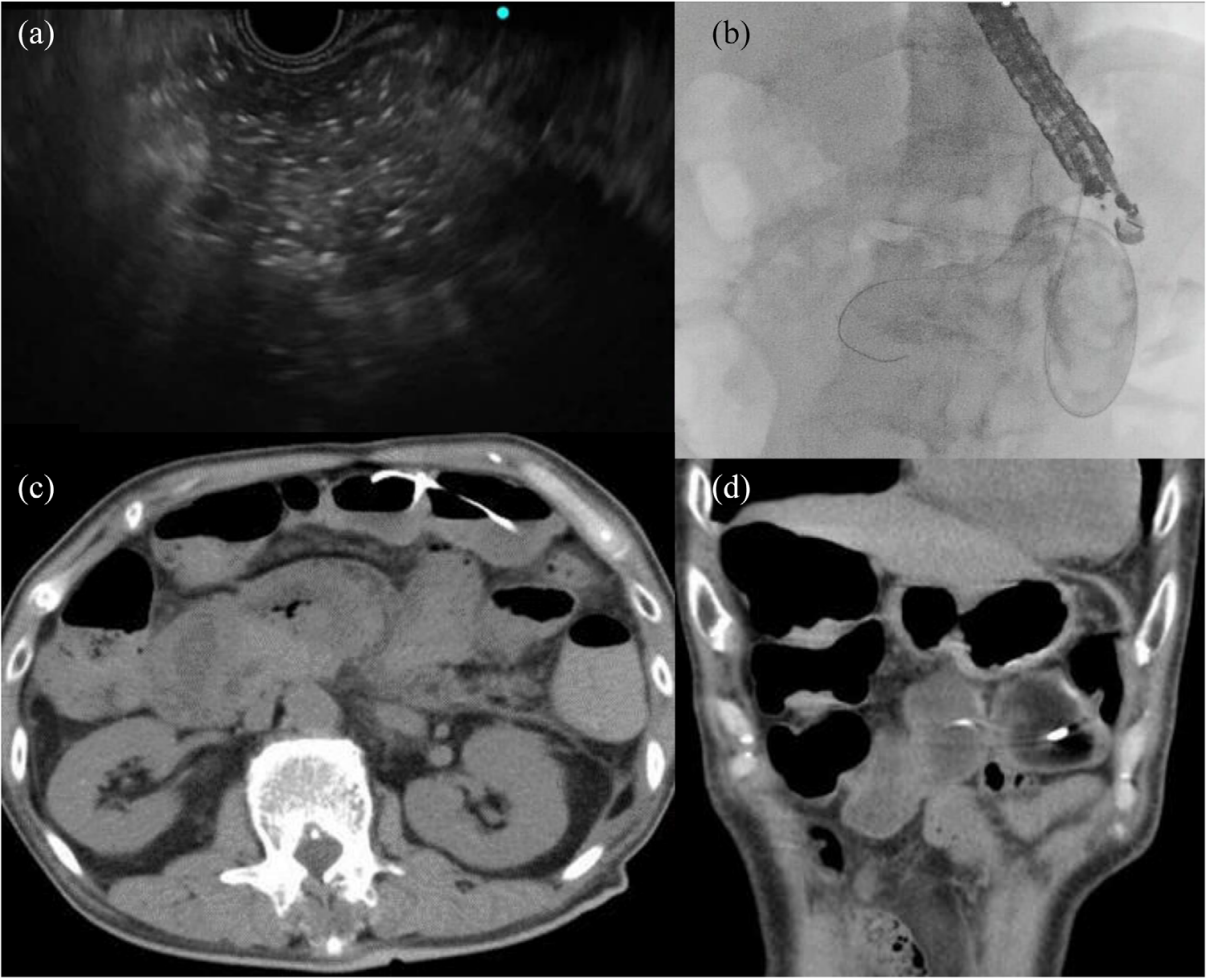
(a–d) EUS‐CD. The cyst (the lumen of the colon was thought to be the cyst) was punctured, and an ENBD tube was placed. The next day, CT showed that the ENBD tube had been placed in the transverse colon, which was an accidental puncture. EUS‐CD, endoscopic ultrasound‐guided cyst drainage; ENBD, endoscopic nasobiliary drainage; CT, computed tomography.
